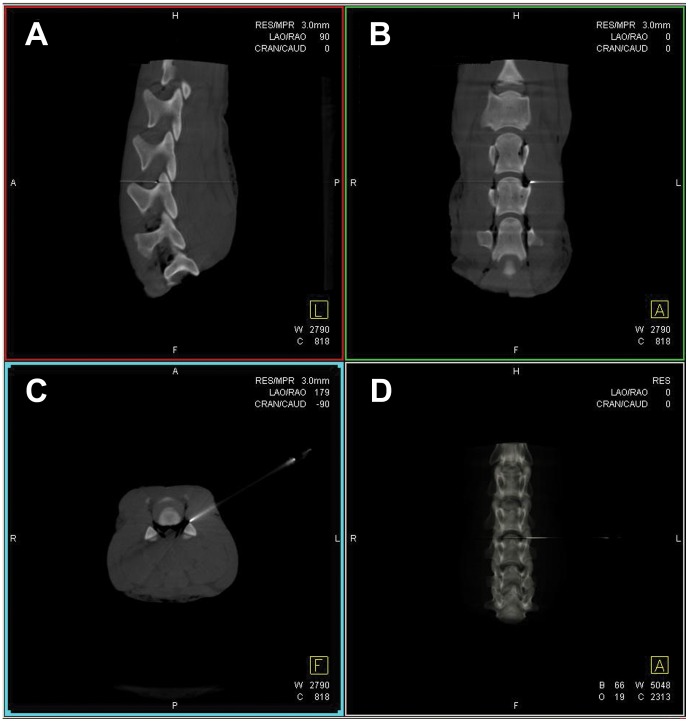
Needle tip localization in post-puncture control Dyna-CT images. Lateral (A), coronal (B), and axial views (C) of the multiplanar reconstructions, as well as (D) three-dimensional rendering of the Dyna-CT of the *ex-vivo* lamb cervical spine-model demonstrating the location of the needle tip and the trajectory used to access the neural foramen. The needle tip is located in the posterior part of the neural foramen, anatomically well away from the vertebral vessels but directly adjacent to the path of the nerve root.
